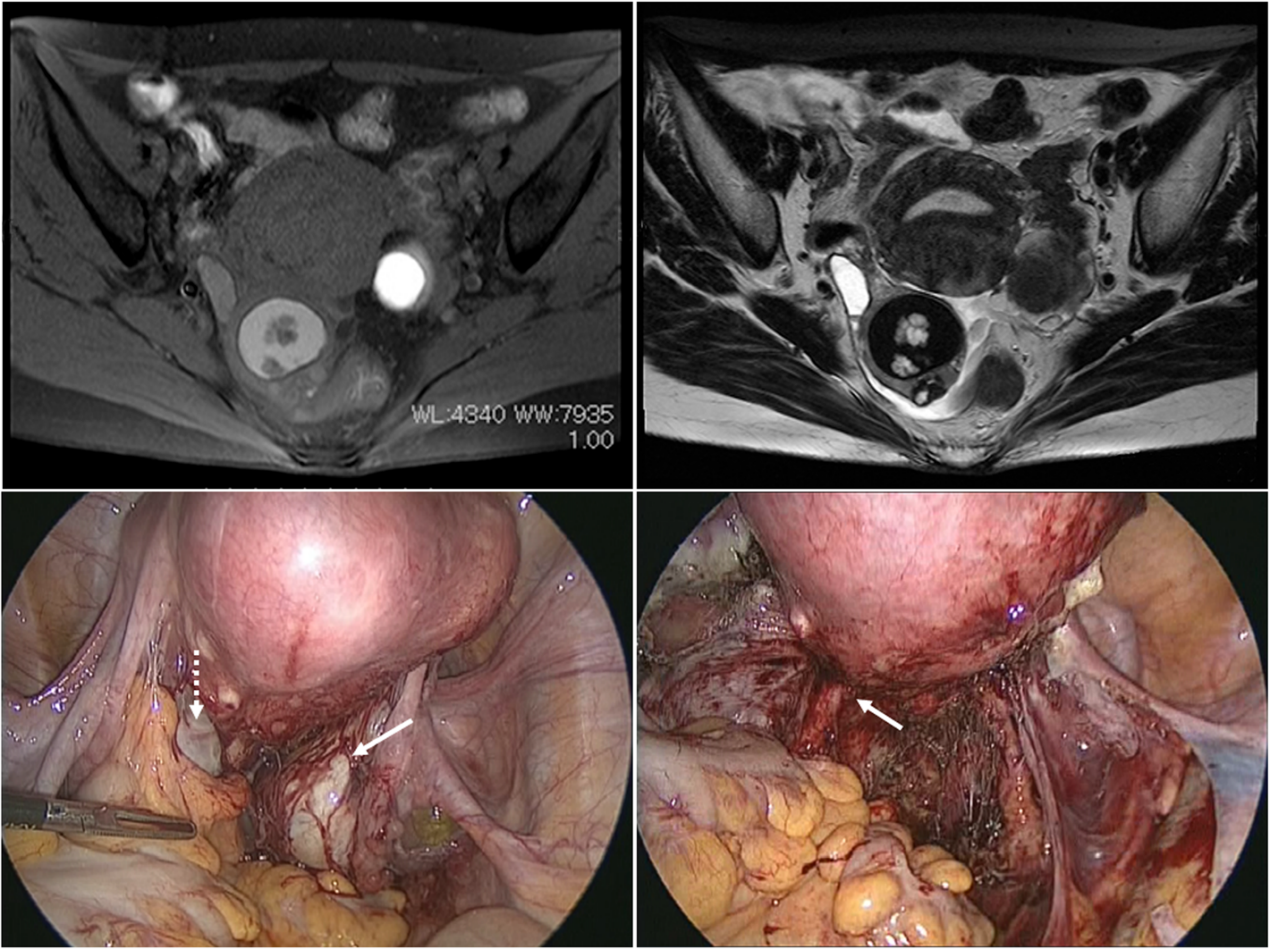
Magnetic resonance images show a right ovarian tumor with a mural nodule and a left ovarian endometrioma. a Transverse T1-weighted fat-saturated image after gadolinium enhancement. b Transverse T2-weighted image. c Laparoscopic findings before surgery of the bilateral ovarian mass with adnexal adhesion. White dotted arrow indicates left ovarian endometrioma, and white solid arrow indicates right ovarian tumor. d Laparoscopic findings after bilateral salpingo-oophorectomy. White arrow indicates lesions suspected as deep infiltrating endometriosis lesions in the left uterosacral ligament
